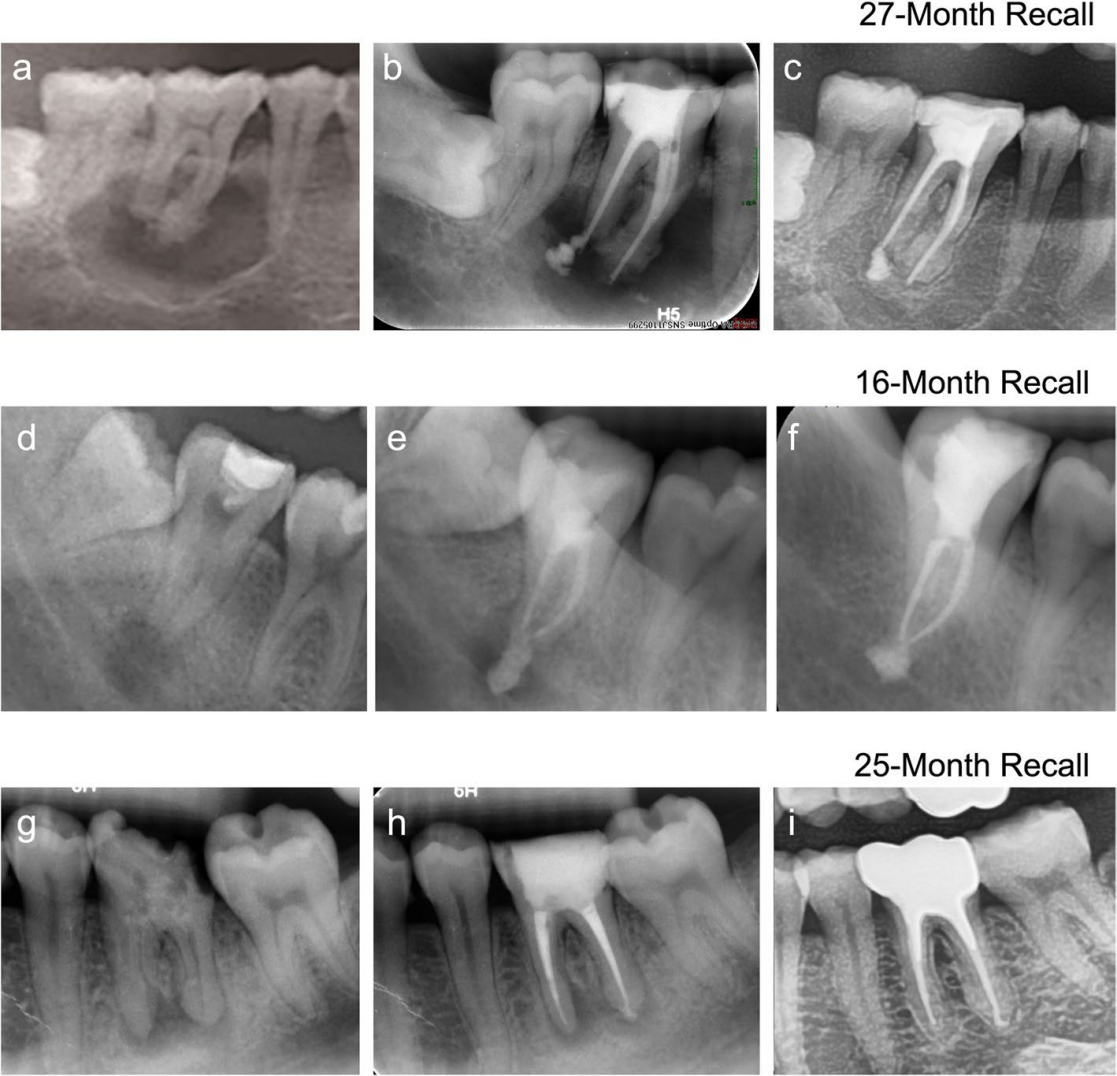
Preoperative (a, d and g), postoperative (b, e and h), and recall (c, f and i) radiographs of the outcomes of healed teeth
